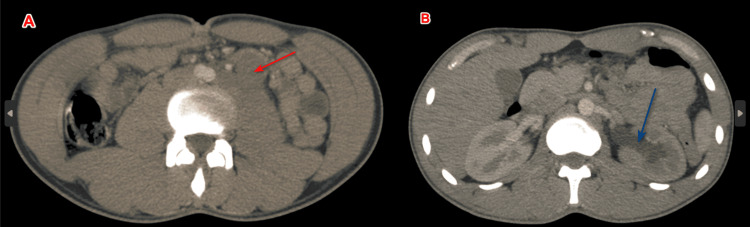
Abdominal and pelvic CT axial section showing left lateral-aortic adenopathy (red arrow) compressing the homolateral ureter (A) and responsible for uretero-pyelo-caliceal dilatation (blue arrow) (B)
